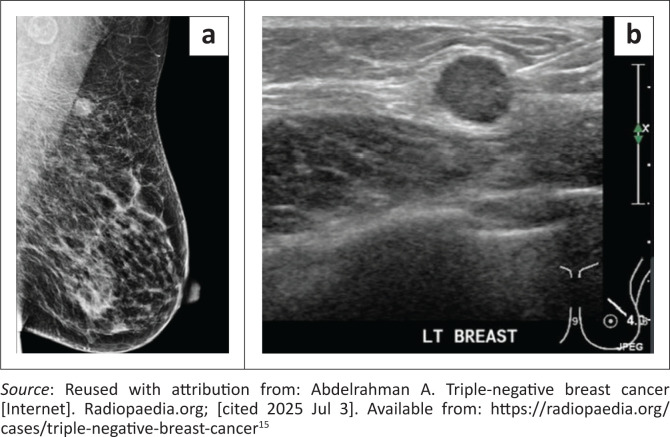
(a) Mediolateral oblique (MLO) mammographic view of the left breast of a 50-year-old female, demonstrating a round, circumscribed intermediate-density mass in the upper outer quadrant with an ipsilateral axillary lymph node. The lesion mimics benign morphology, although such roundness can be more common in triple-negative breast cancer (TNBC) than fibroadenoma.^15^ (b) Ultrasound image of the left breast in the same patient showing a round, circumscribed, hypoechoic mass with posterior acoustic enhancement. While this morphology resembles a benign fibroadenoma, the round shape and the presence of subtle microlobulated margins should raise suspicion for malignancy—features more commonly seen in TNBCs than in true fibroadenomas.^15^
